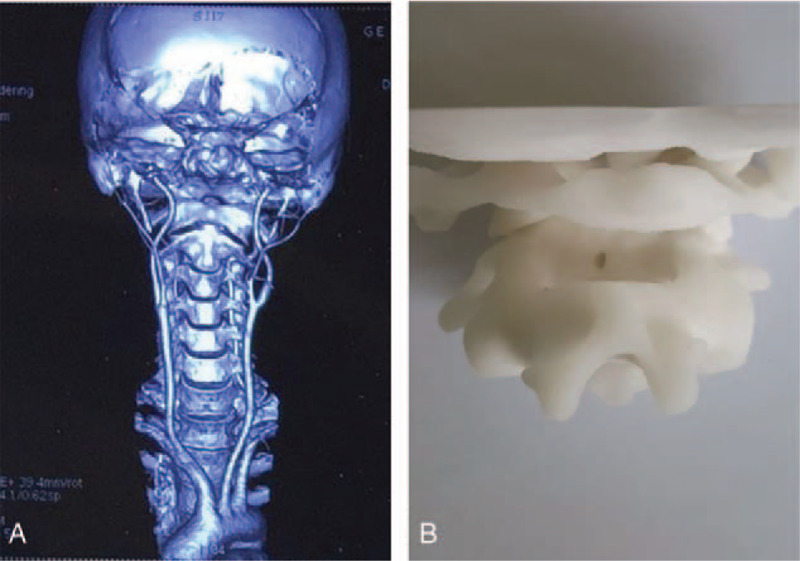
A. Three-dimensional CTA of the cervical spine shows the relationship between blood vessels and the vertebral spine; B. Printing the atlantoaxial spine shows the anatomy of the upper cervical spine. CTA = computed tomography angiography.
